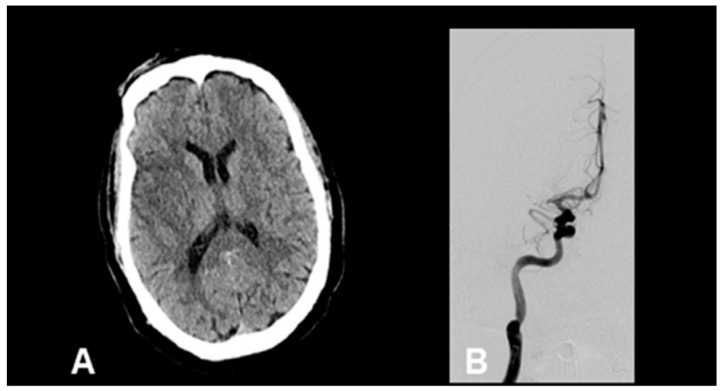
Representative neuroimaging from a patient with recent SARS-CoV-2 infection, new-onset atrial fibrillation, and proximal right MCA occlusion. (A) Non-contrast head CT reveals areas of hypoattenuation within the right MCA vascular territory. (B) Conventional angiography revealing persistent proximal M1 occlusion. Adapted with permission from Batra, A.; Clark, J.R.; LaHaye, K.; Shlobin, N.A.; Hoffman, S.C.; Orban, Z.S.; et al. (2021) [57].
